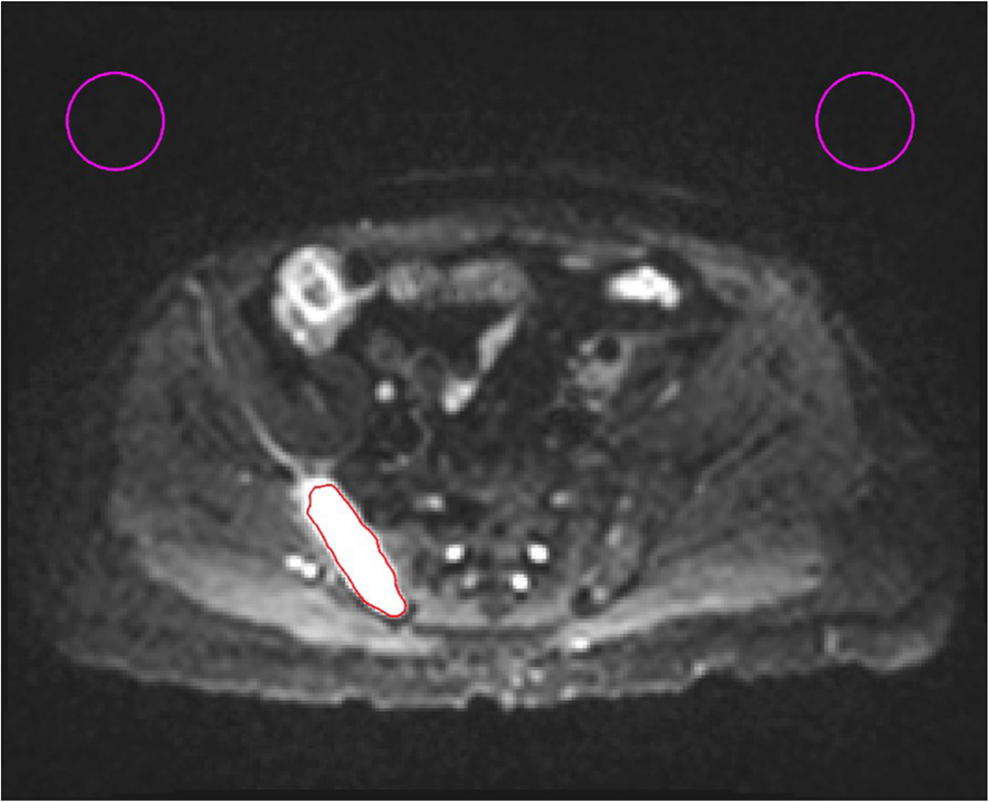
An example of the ROIs drawn (pink) on DWI *b* = 50 s/mm^2^ image to estimate the noise. The lesion is delineated in red. The number of pixels in the ROIs was kept constant across the different matrix sizes used
